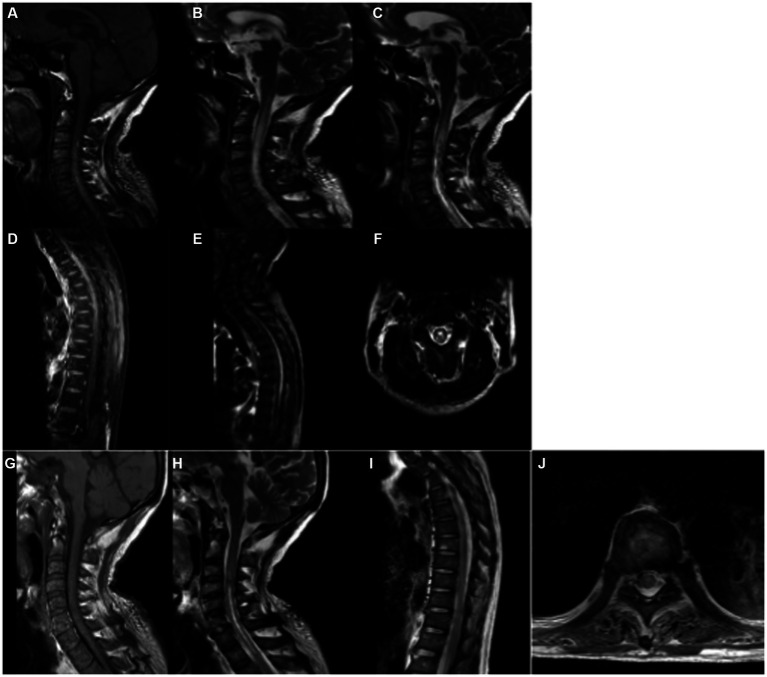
MR Imaging of *Paragonimus* Infection in the Spinal Cord of the Patient. Sagittal T1-weighted imaging (T1WI) (A) and T2-weighted imaging (T2WI) (B) show long segment abnormal signals parallel to the C1-T1 spinal cord levels. On T1WI, the signals are mostly isointense, while on T2WI, they appear hyperintense. Axial T2WI (F) reveals a high-intensity “figure-eight” sign, with a slightly lower signal ring-shaped cyst wall and a central area of high-signal necrotic tissue. Sagittal T1WI (D) and T2WI (E) demonstrate abnormal enhancement along the T1-T10 spinal cord levels, showing strip-like low-intensity signals within the area of abnormal enhancement. After 20 days of treatment, a follow-up MRI of the cervical and thoracic spine showed that in panels (G–J), the inflammatory edema signals in the spinal cord had reduced or disappeared to varying degrees.
